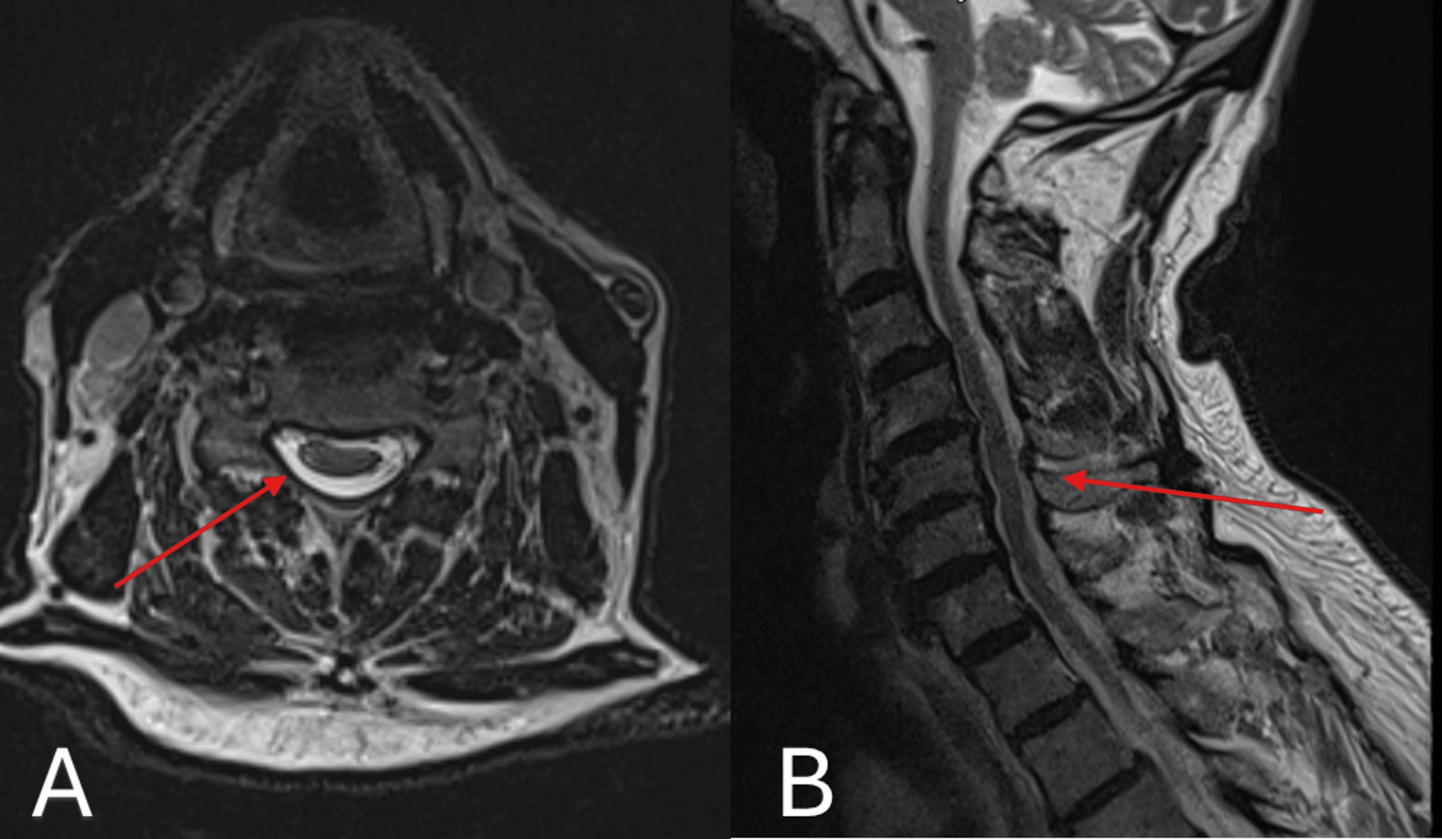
Axial T2-weighted MRI (A) showing resolution of the spinal epidural haematoma, and sagittal T2-weighted MRI (B) demonstrating residual, though reduced, myelopathic signal changes at the C4-C5 level. MRI, magnetic resonance imaging
